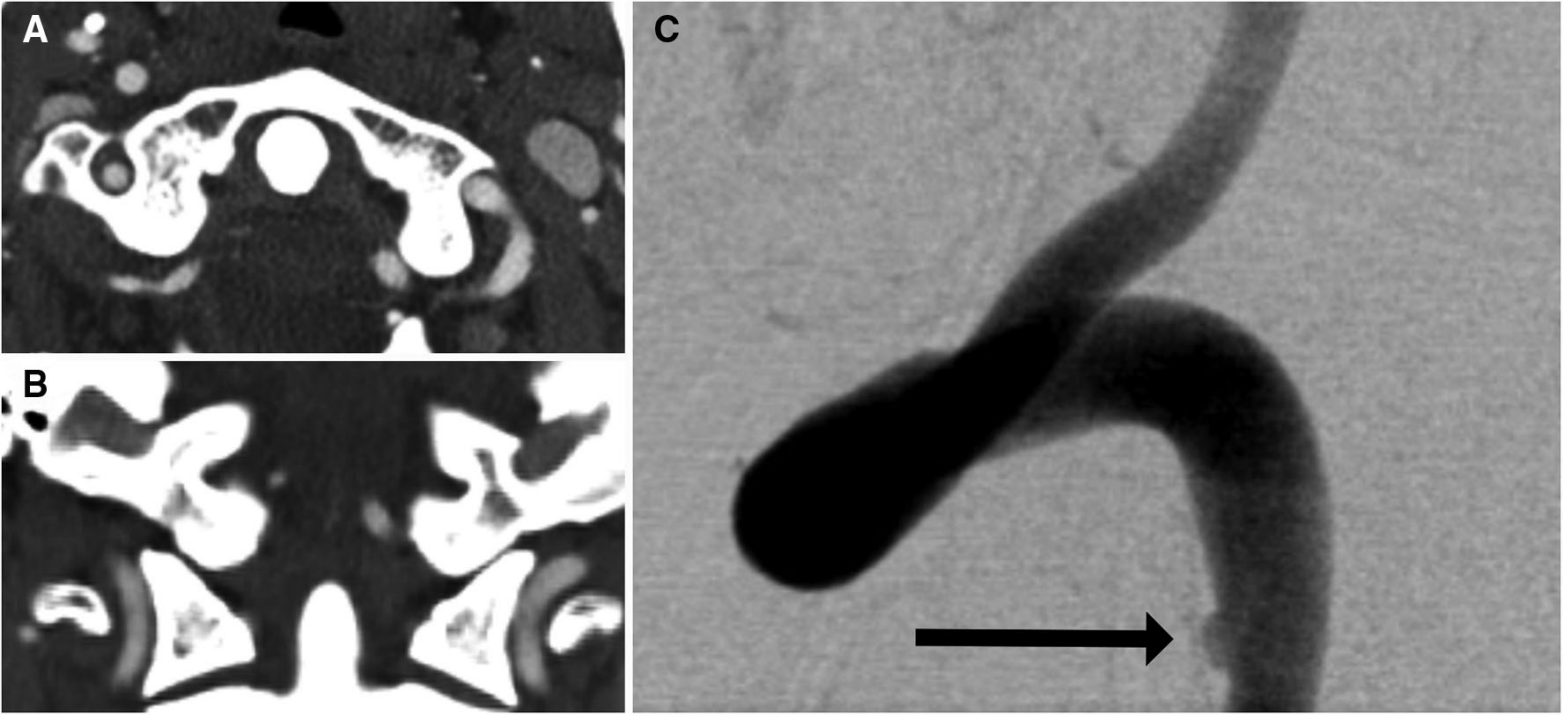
Imaging of a 35-year-old man who presented after a motor vehicle crash. Initial axial (A) and coronal (B) CTA were negative for vertebral artery dissection. Because of a high level of suspicion for vertebral artery injury, DSA (C) was performed. DSA showed subtle intimal injury involving the left vertebral artery (arrow).
